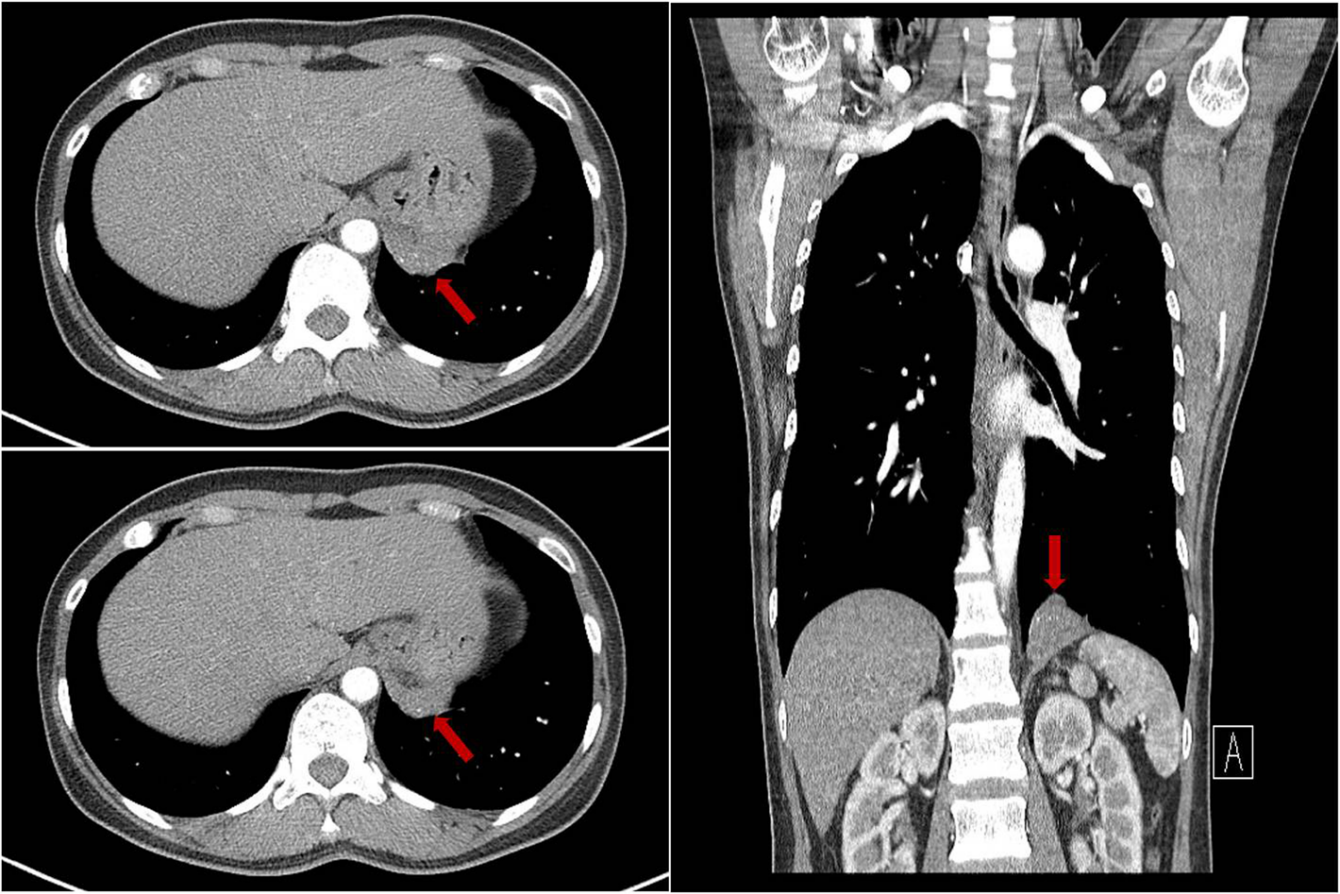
Preoperative chest computed tomography findings.
